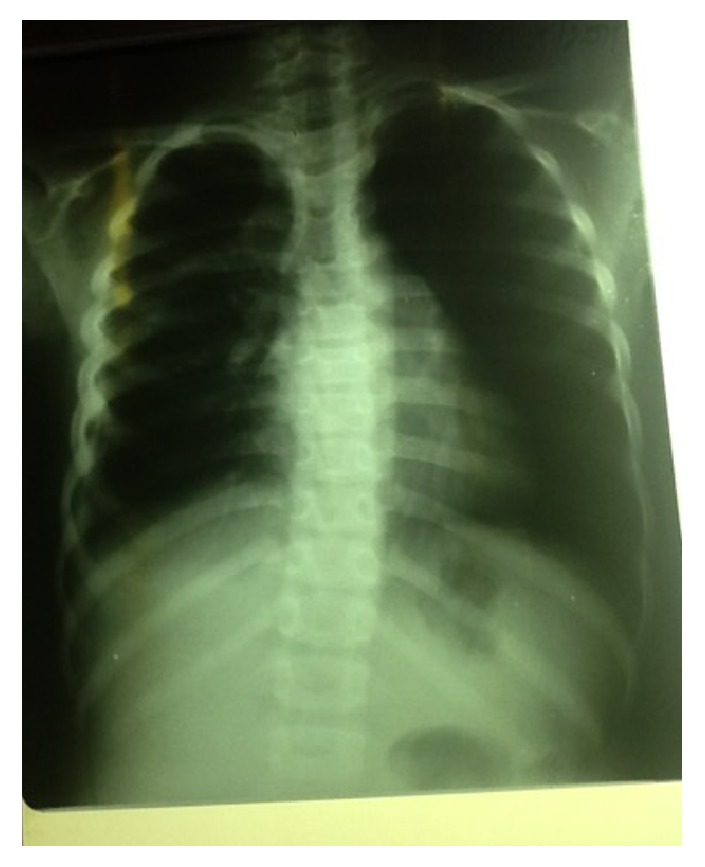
Chest X-ray: control at one year after both surgeries in the 6 Y/O girl.
